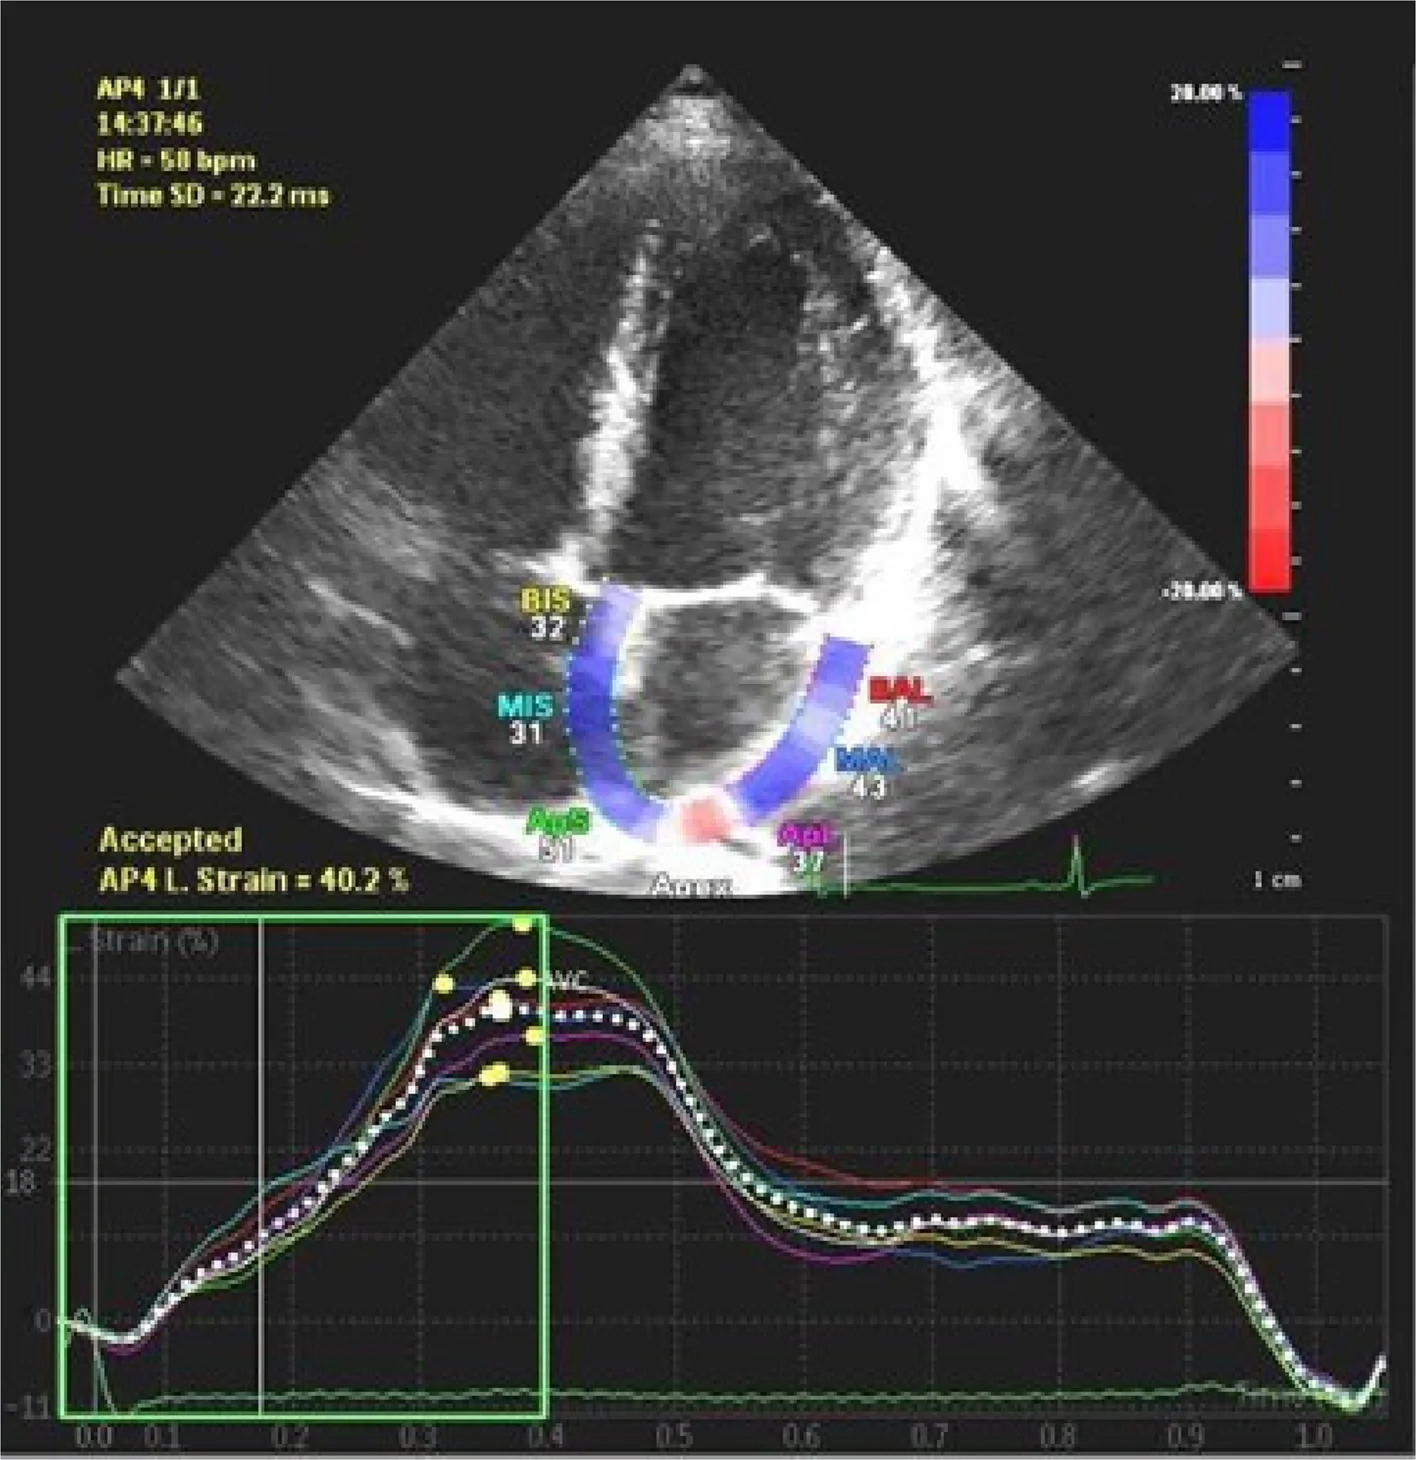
Speckle-tracking echocardiography of the left atrium. AP4, apical four-chamber; ApL, apical anterolateral; ApS, apical inferoseptal; BAL, basal anterolateral; BIS, basal inferoseptal; bpm, beats per minute; HR, heart rate; MAL, mid anterolateral; MIS, mid inferoseptal; ms, milliseconds; SD, standard deviation
